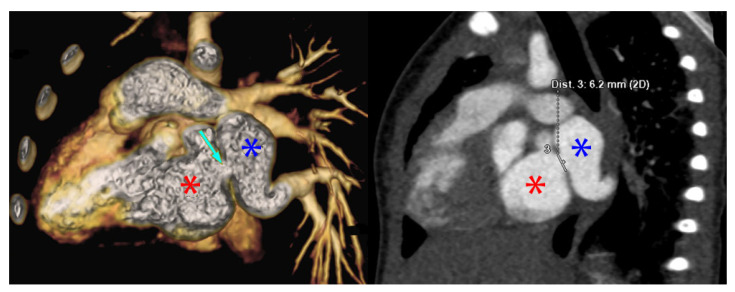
CT scans from the above described patient from Malaysia with repaired supracardiac TAPVR with recurrent PVS. The image on the left is a 3D reconstruction of a CT angiogram. The blue star denotes the pulmonary vein confluence, the red start denotes the left atrium. The light blue arrow on the left image denotes the area of stenosis. The image on the right that of the traditional CT angiogram with blue and red stars denoting the pulmonary veins and left atrium, respectively. The line measures the stenosis at 6.2 mm. Cross sectional imaging such as this allows institutions to risk stratify cases and offer surgery to those patients with the best predicted outcome.
